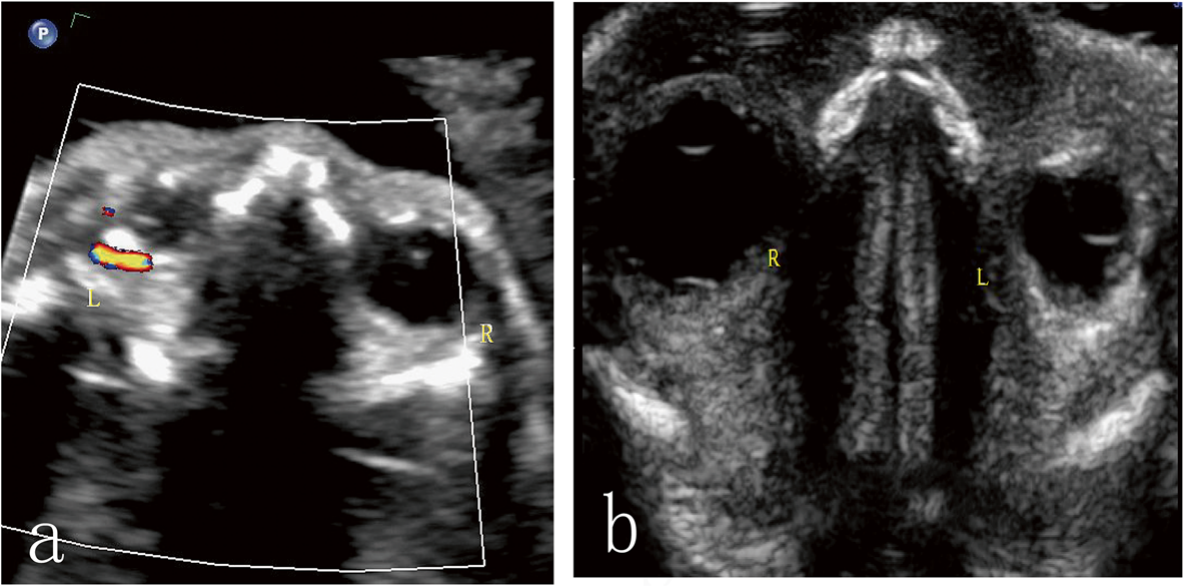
Prenatal sonography in the transverse plane at 24 + 1 weeks of gestation. The left eye globe was much smaller than the right eye globe. Colour Doppler imaging showed blood flow signals in the lesion (a). Using high-frequency ultrasound, a hyperechoic band was observed in the retro-orbital space (b)
